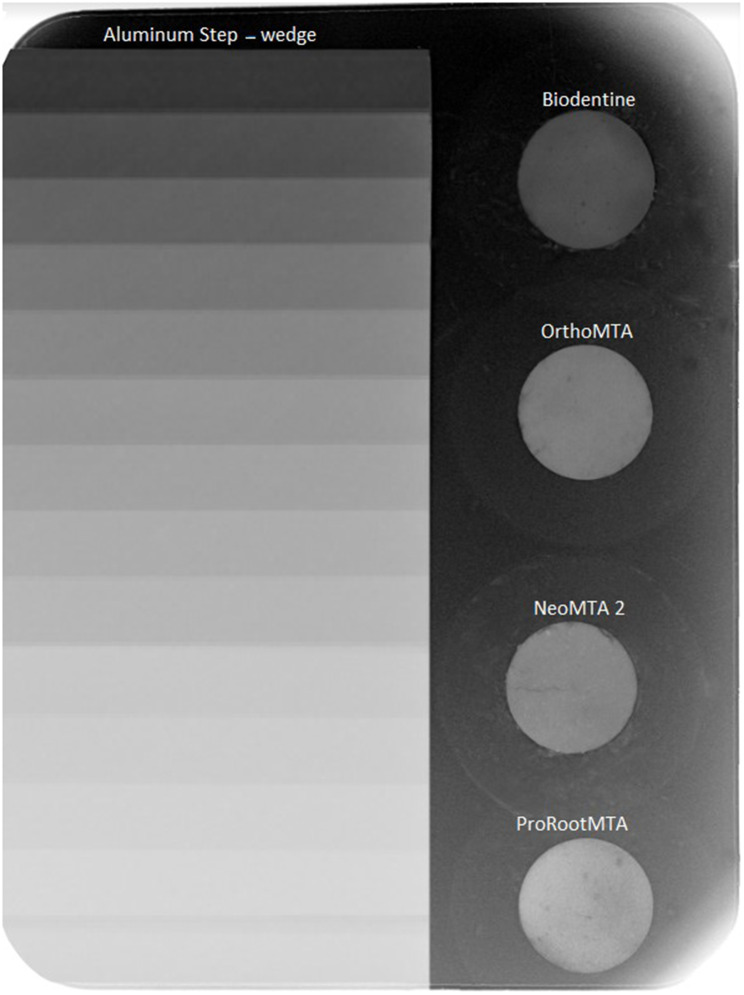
Digital radiographic image of root calcium silicate cement samples and aluminum step-wedge
